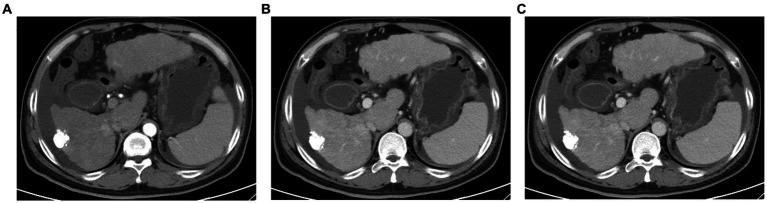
Abdominal enhanced computed tomography images (A) Plain CT scan; (B) arterial phase of contrast-enhanced CT; (C) portal vein phase of contrast-enhanced CT. CT examination of the abdomen show no biliary obstruction and disease progression of intrahepatic lesions.
